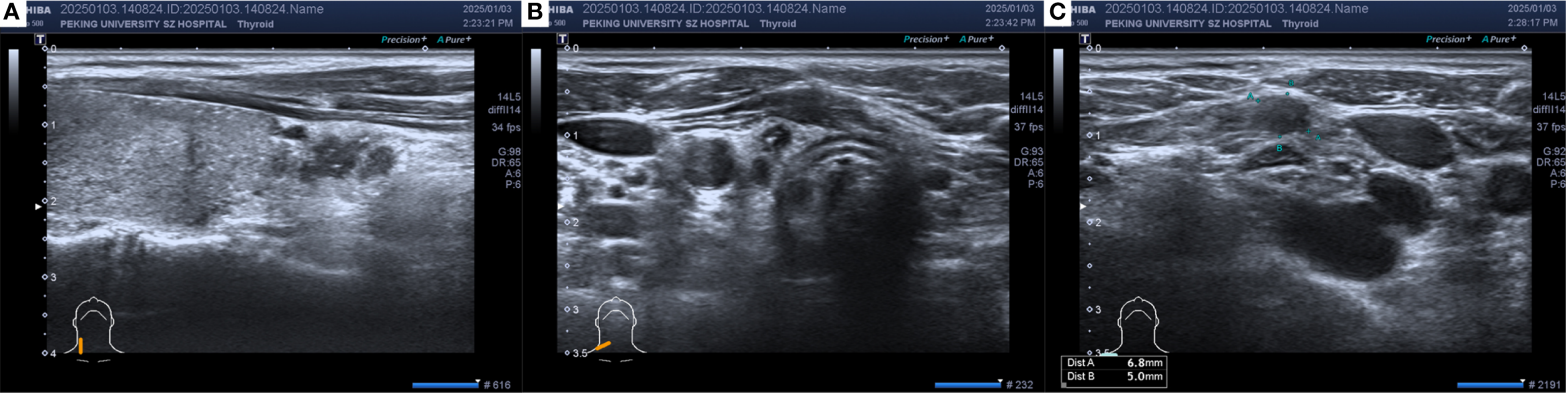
The thyroid ultrasound in December 2024: (A) Hypoechoic thyroid nodule located in the right thyroid lobe. (B) Enlarged lymph node in the central neck region (Level VI). (C) Enlarged lymph node in the right supraclavicular region. Heterogeneous echogenicity in the right thyroid lobe, with multiple scattered punctate hyperechoic foci. A hypoechoic nodule measuring approximately 13×4×8 mm with an irregular shape and punctate hyperechoic foci inside was observed in the lower part of the right lobe. Multiple hypoechoic nodules with irregular shapes, clear boundaries, cortical thickening, and loss of the lymph node hilum structure were found from level IV of the right neck to the right supraclavicular region, with a few hyperechoic foci inside.
